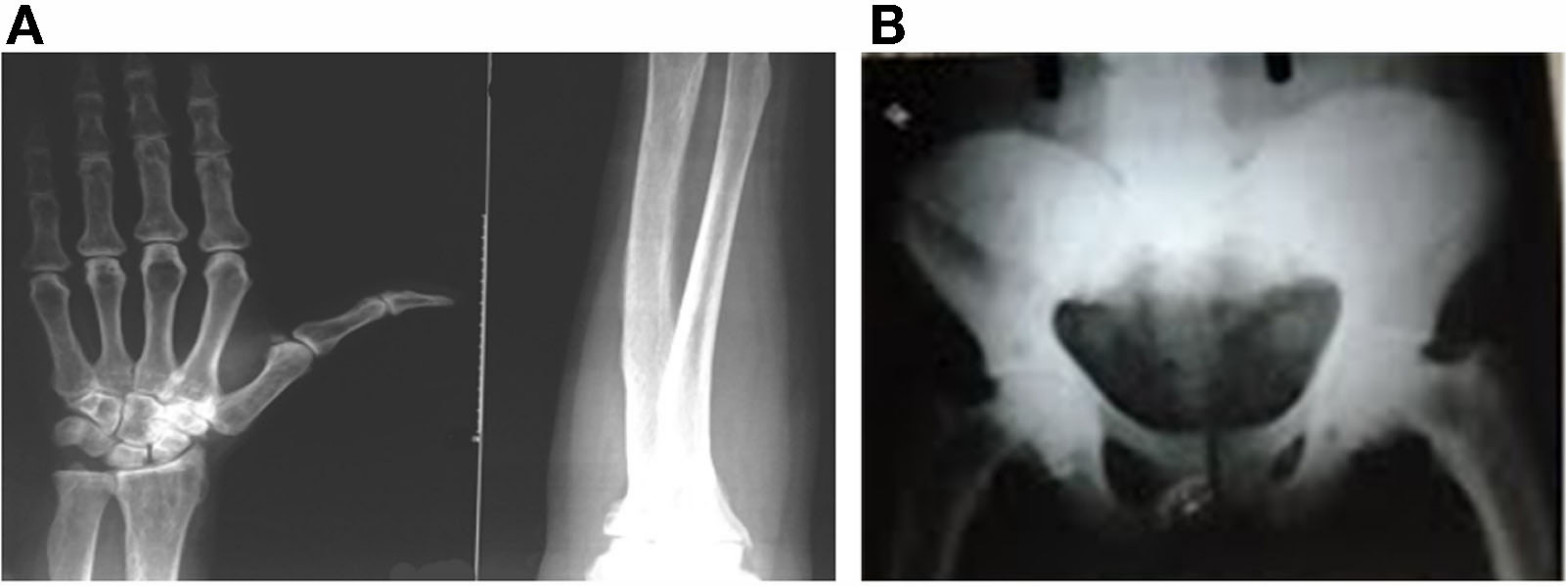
X-ray, (A) forearm showing interosseous membrane calcification, (B) X-ray hip showing ectopic calcification.
